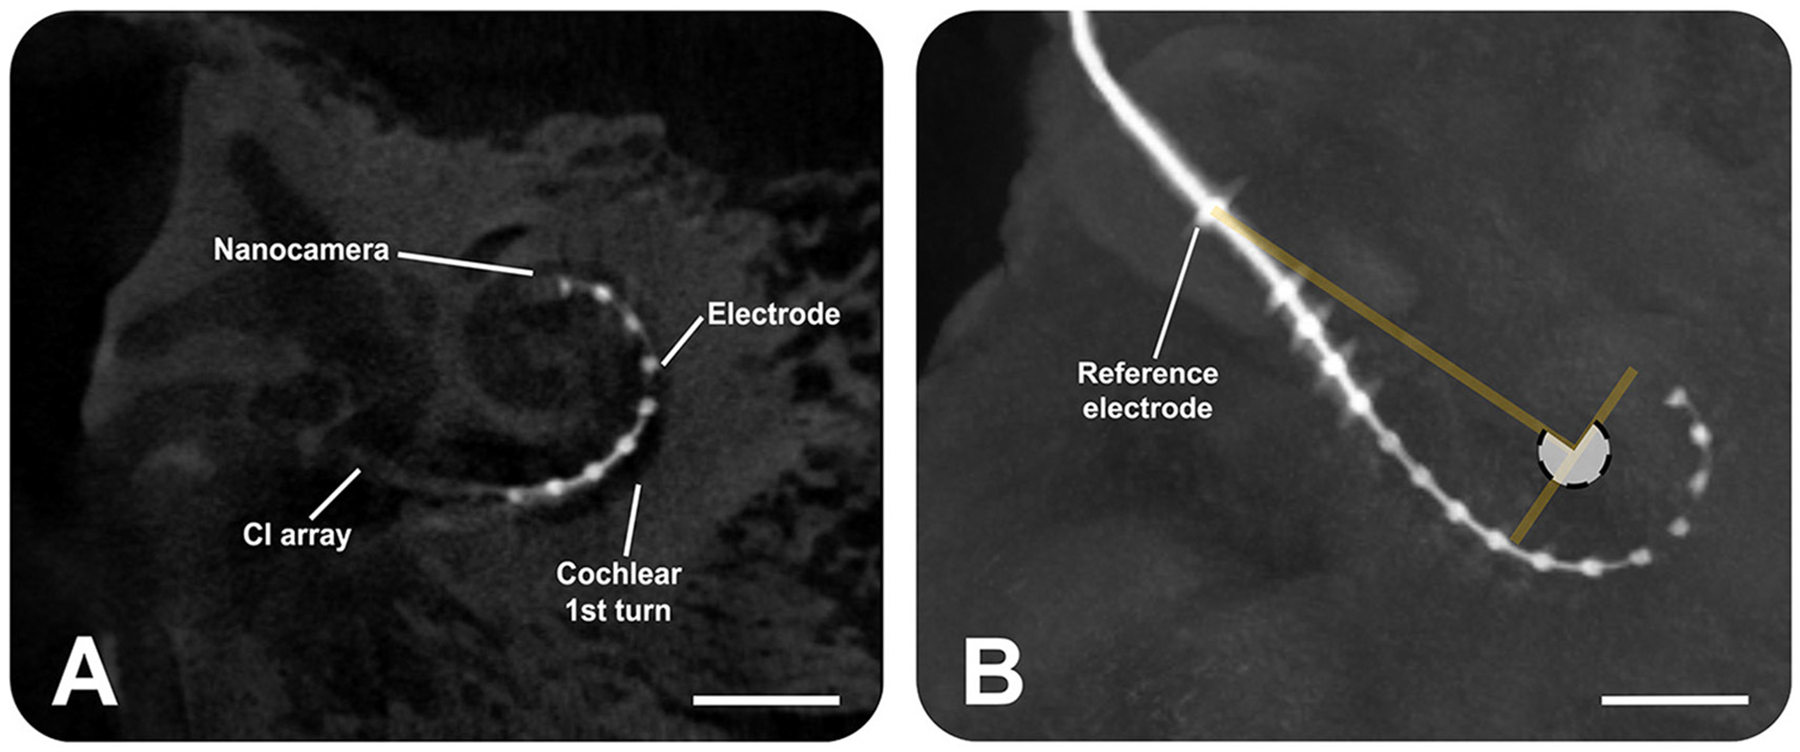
Micro-computed tomography scans show the insertion of the cochlear implant electrode array (Advanced Bionics) equipped with the nanocamera into the human cochlea. (A) Sagittal slice, providing a cross-sectional view. (B) Sagittal view from the three-dimensional volume rendering of the human temporal bone, highlighting the electrode. The angular insertion depth is ~270° as the white transparent circle shows. The scale bars represent 4 mm.
